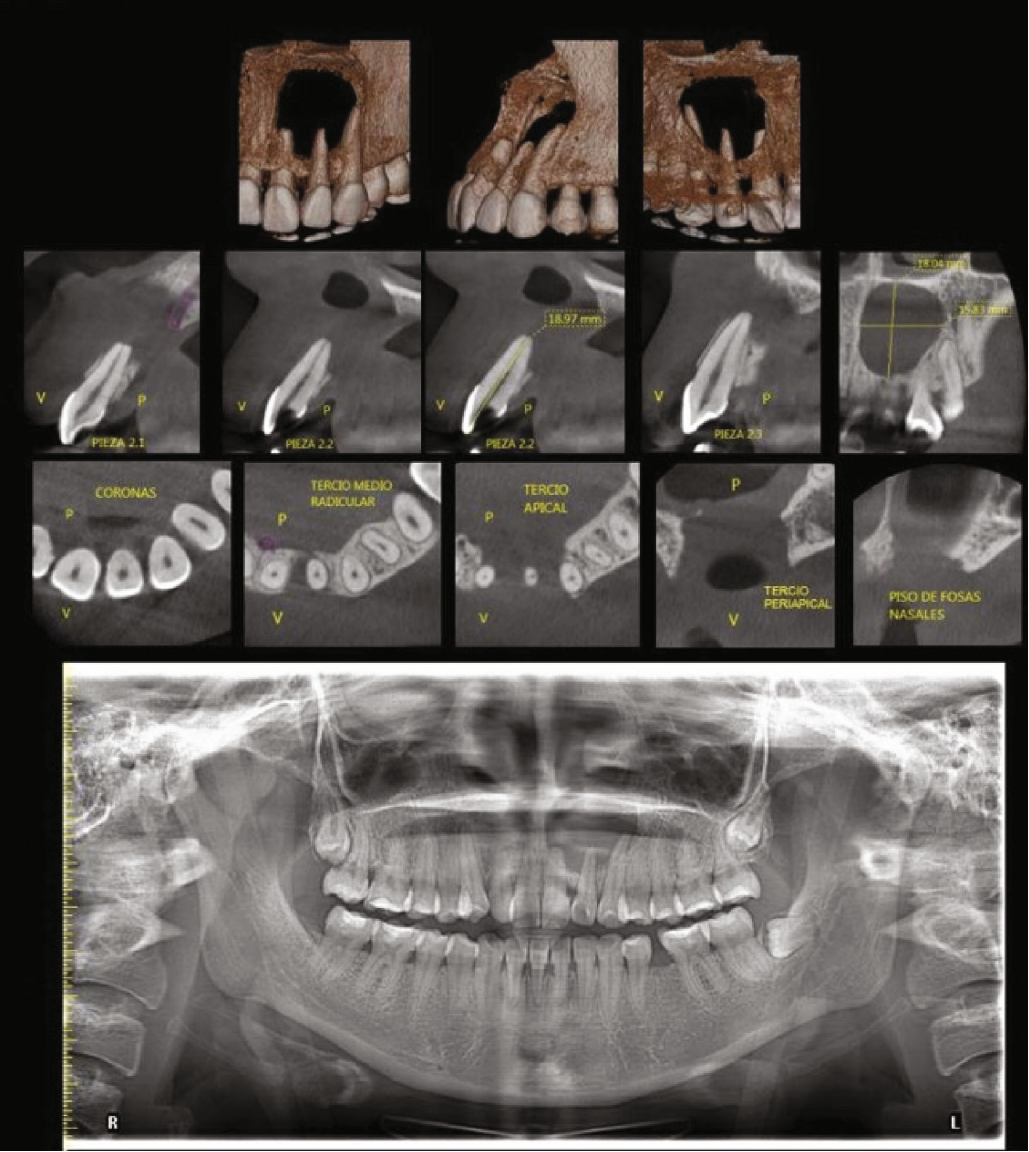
Initial CBCT study showing large osteolytic lesion.
